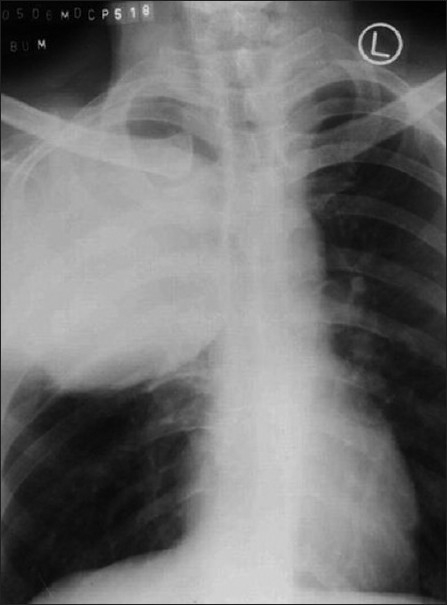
Chest radiograph showing the right mediastinal tumor
